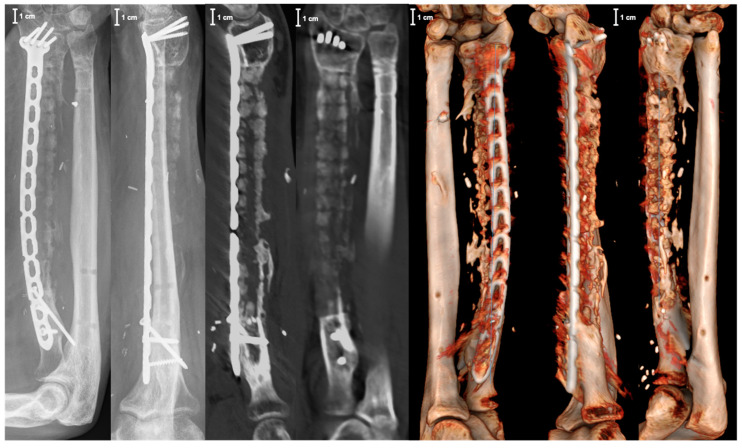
X-ray images (AP and lateral view) and computer tomography (CT) of the right forearm 3 months after surgical intervention.
